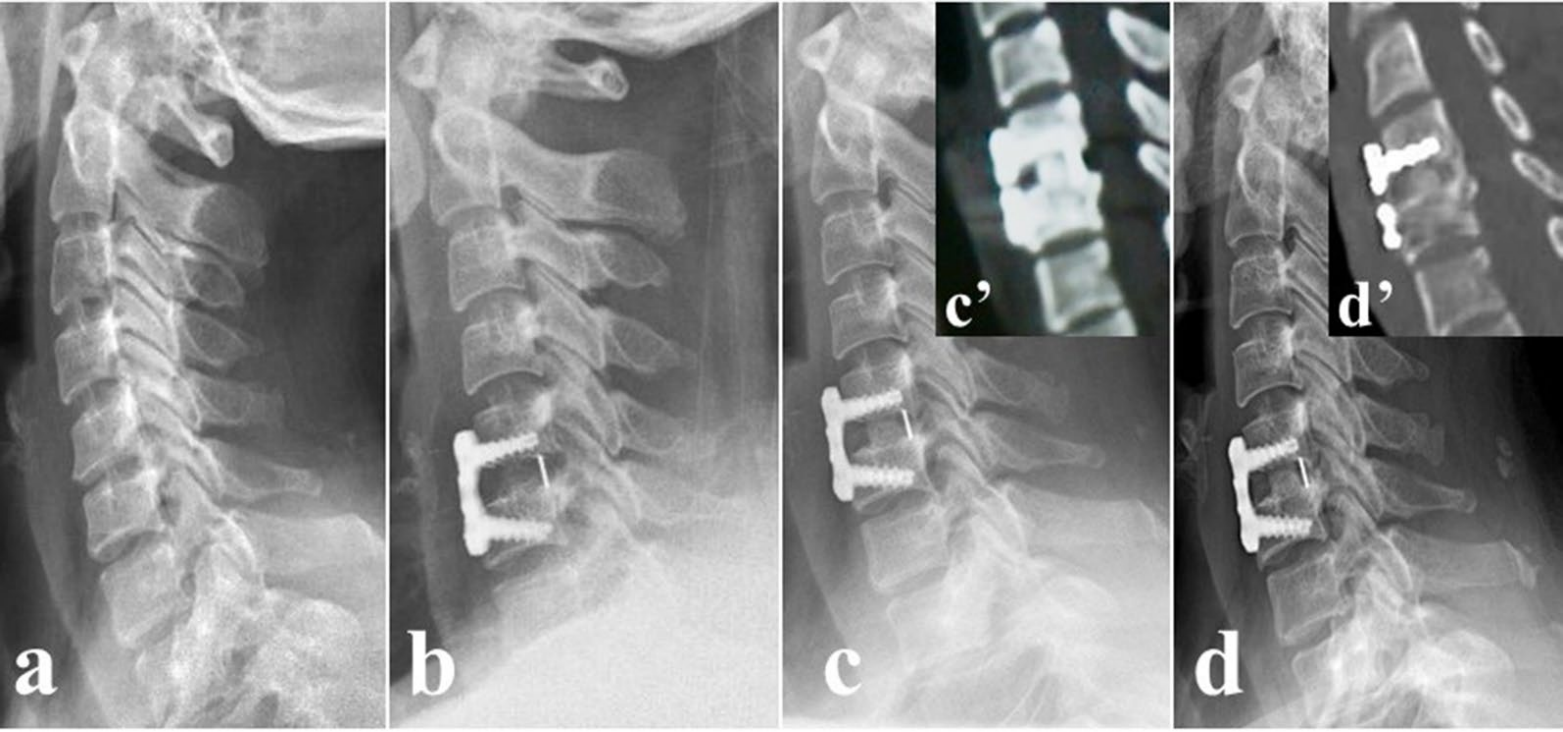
A 46-year-old woman undergoing anterior cervical discectomy and fusion surgery with a PEEK/Ti/HA cage. a Preoperative plain lateral radiographs image. b Postoperative lateral radiograph image. c Lateral radiograph image at 3-month follow-up. c’ CT scans at 3-month follow-up. d Lateral radiograph image at the final follow-up. d’ CT scans at the final follow-up. PEEK, polyetheretherketone; Ti, titanium; HA, hydroxyapatite; CT, computerized tomography
